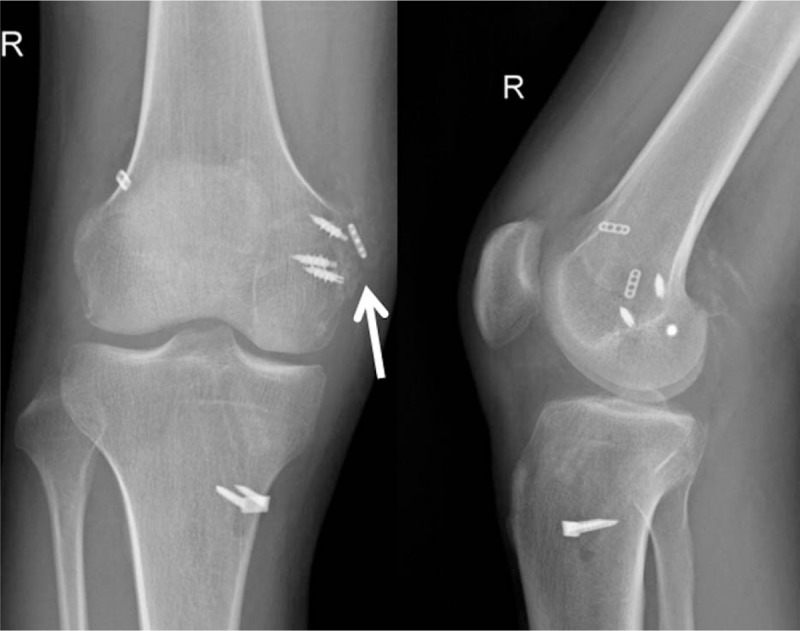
Anteroposterior (AP) and lateral radiographs at 3-month follow-up. Arrow indicates mature heterotopic ossification at the medial side of the knee.
